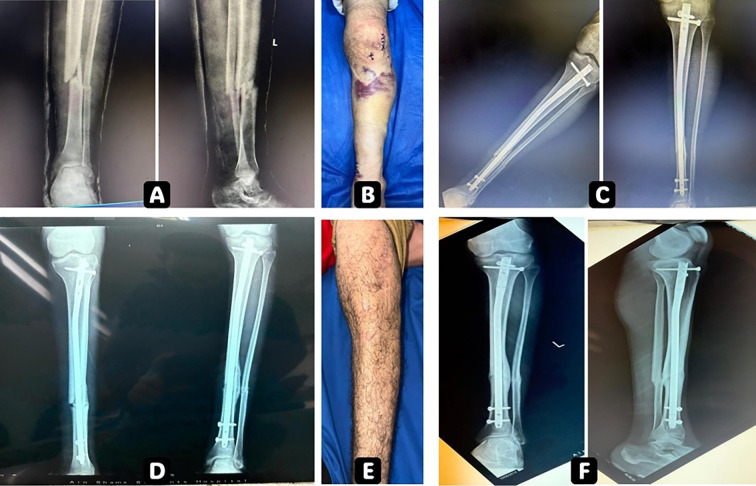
Representative case managed with IMN via the LPP: A) Preoperative anteroposterior and lateral radiographs of a left tibial shaft fracture. B) Clinical photograph showing the first postoperative dressing. C) Immediate postoperative anteroposterior and lateral radiographs. D) Three-month follow-up anteroposterior and lateral radiographs. E) Clinical photograph at 6 months showing the surgical wound. F) Six-month follow-up anteroposterior and lateral radiographs.
